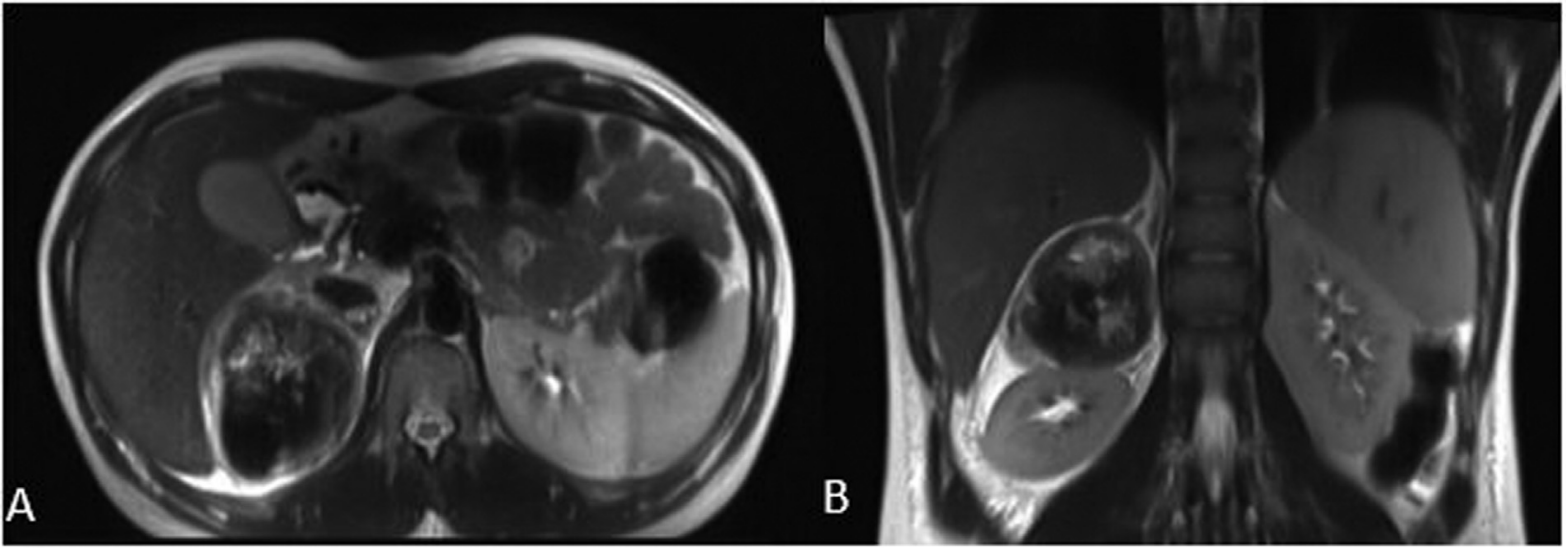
(A) Axial view and (B) coronal view of magnetic resonance imaging T2-weighted images showing a well-defined heterogenous hypointense lesion in the lateral limb of right adrenal gland.
